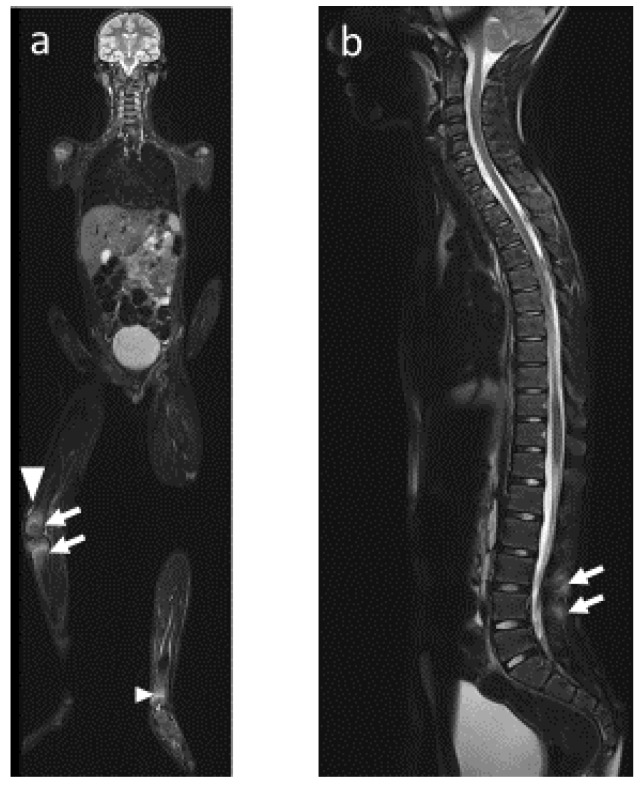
Whole body MRI, same patient 18 months later, CRMO with probable overlapping SpA. Asymmetrical position during MRI acquisition due to intense pain. Coronal plane in (a) STIR-weighted sequence showing bone marrow edema in the right lower femur and right upper tibia (arrow), associated with joint effusion (arrowhead) and bone marrow edema in the metaphysis of the left lower tibia (small arrowhead). Sagittal plane in (b) STIR-weighted sequence showing periosseous edema in the interspinous space (arrows).
